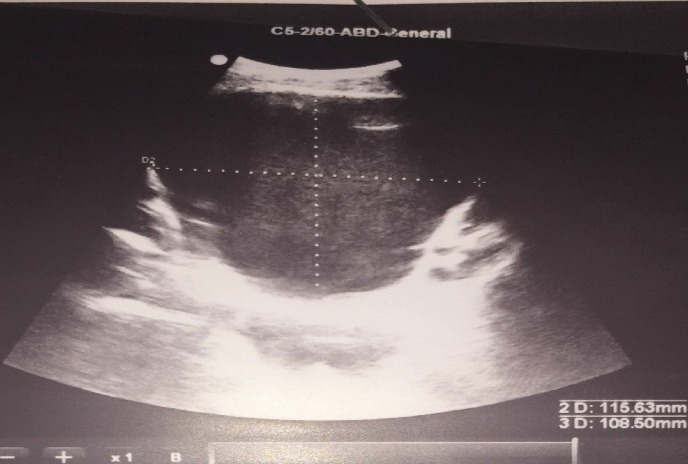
ultrasonographic imaging of the abdomen and pelvis (coronal section): huge cystic lesion
